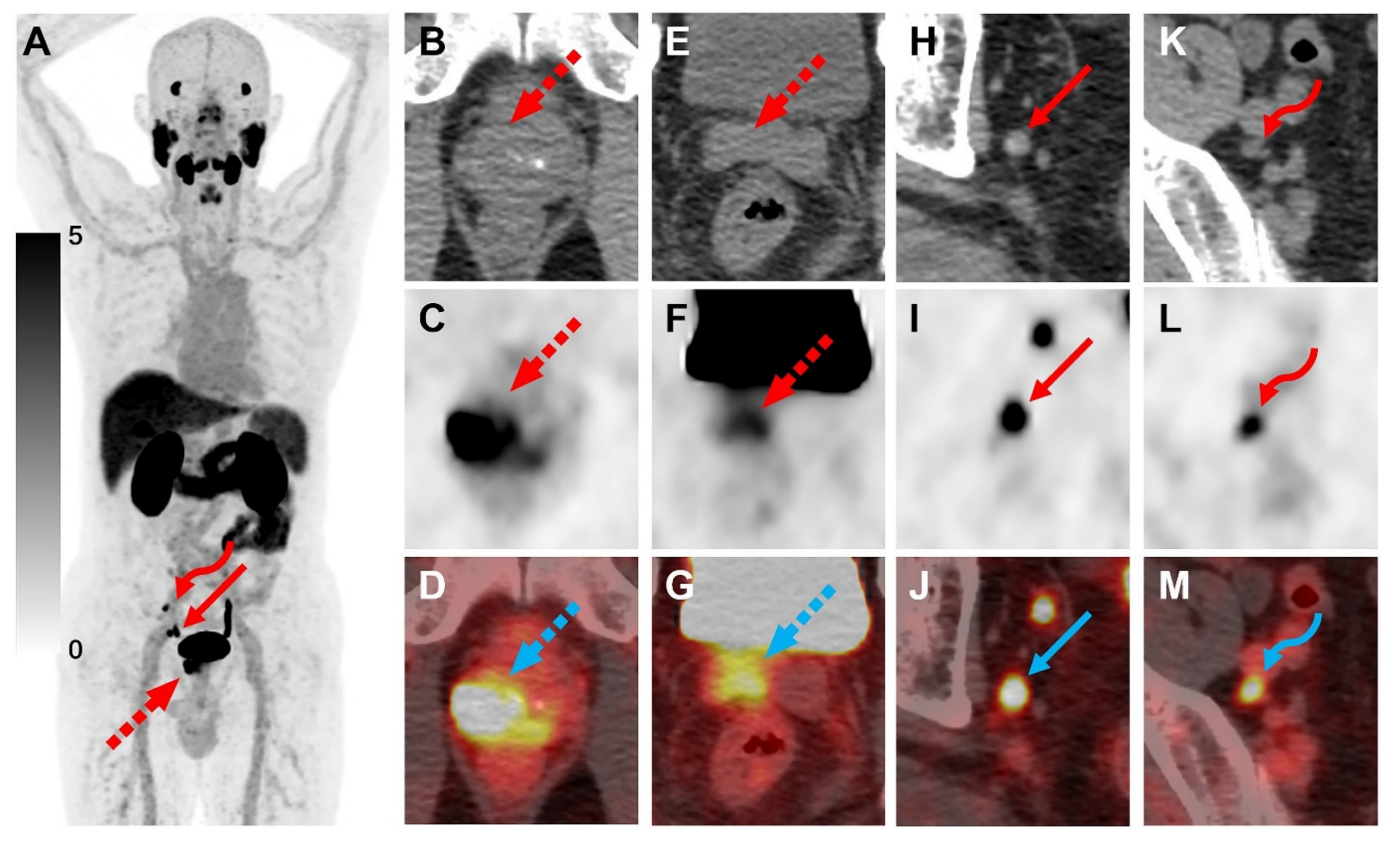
A 67-year-old man diagnosed with PCa, with a Gleason score of 4+5=9 and a total PSA level of 7.35 ng/mL, underwent [^18^F]AlF-Thretide PET/CT. (A) The MIP image revealed the primary PCa lesion (dashed arrow) as well as two lymph node metastases (arrow and curved arrow) in the pelvic region. (B-G) The scan highlighted the primary lesion's invasion into the right seminal vesicle. (H-M) The axial CT showed two small lymph nodes with short axes of 0.8 cm and 0.5 cm, respectively. Corresponding PET and fusion images demonstrated SUVmax of 7.9 and 10.3 for the lymph nodes. The smaller lymph node was missed by pelvic MRI. Both lymph node metastases exhibited a significant reduction in volume after ADT.
